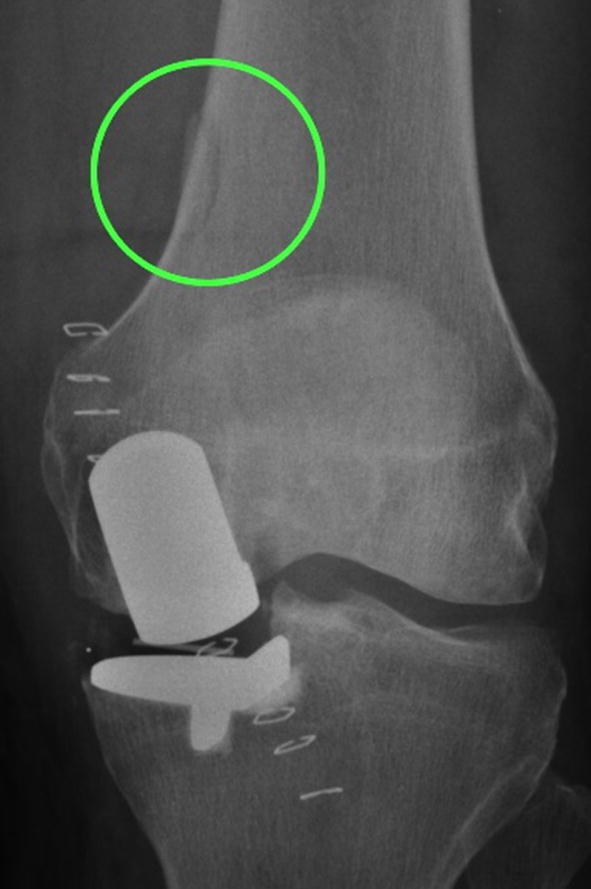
A post-primary anteroposterior radiograph demonstrating a malaligned femoral component, which is likely due to a malpositioned intramedullary guide rod that has pierced the femoral cortex (circled)
